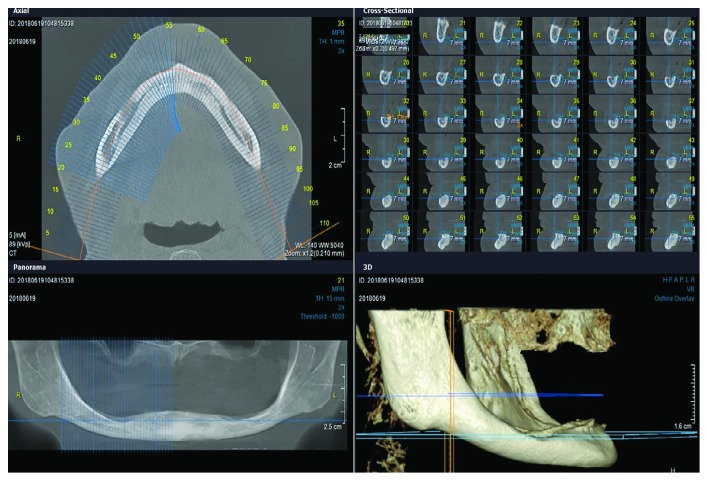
A CBCT scan of one patient with extensively resorbed mandibular alveolar ridge.
